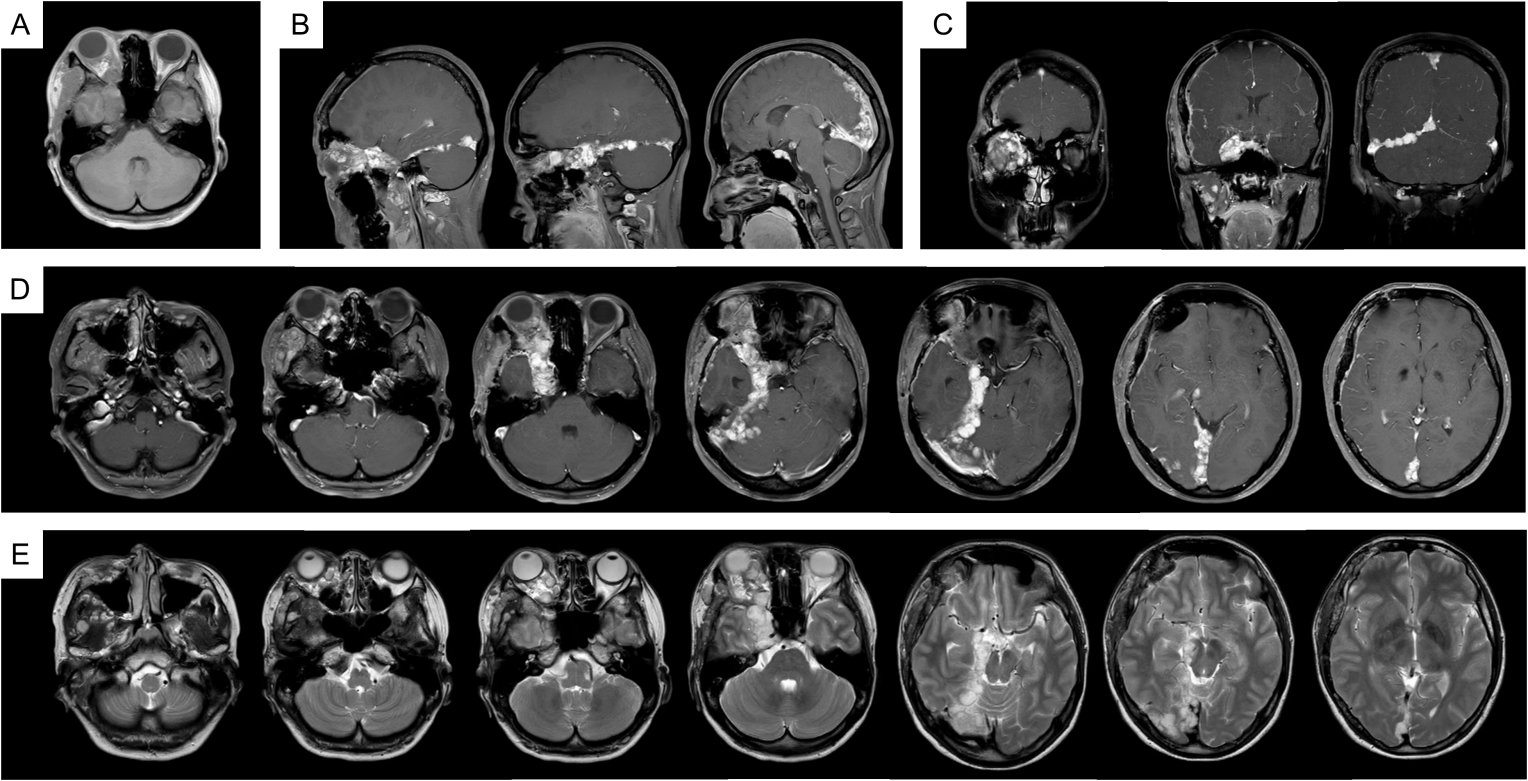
MRI imaging after the palliative surgery. (A) T1WI; (B) T1WI-C coronal view; (C) T1WI-C sagittal view; (D) T1WI-C; (E) T2WI-TSE.
